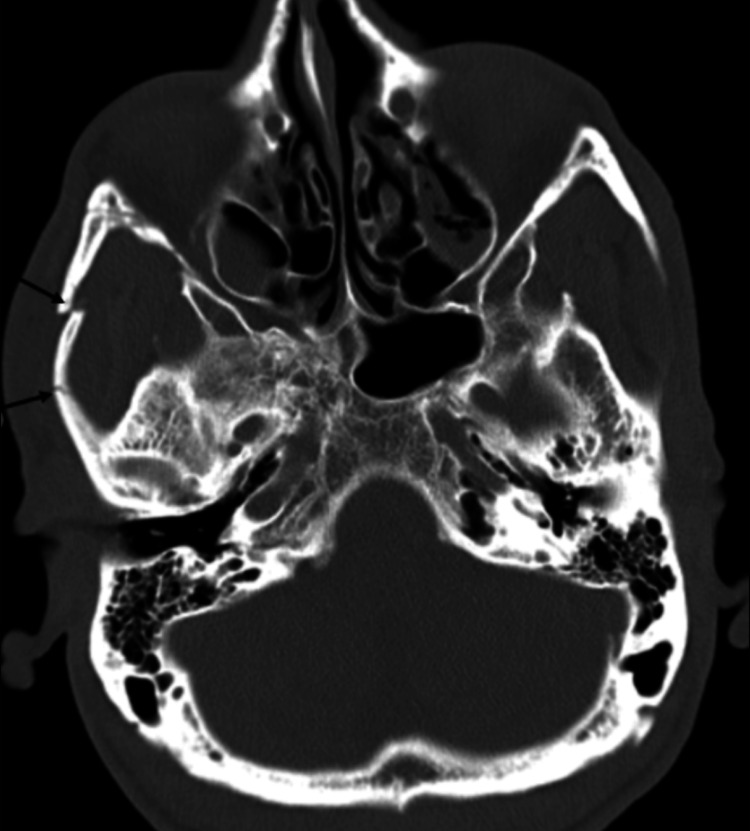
Non-contrast-enhanced CT maxillofacial shows an area with two segmental fractures of the right zygomatic arch (single arrows) CT: computed tomography
